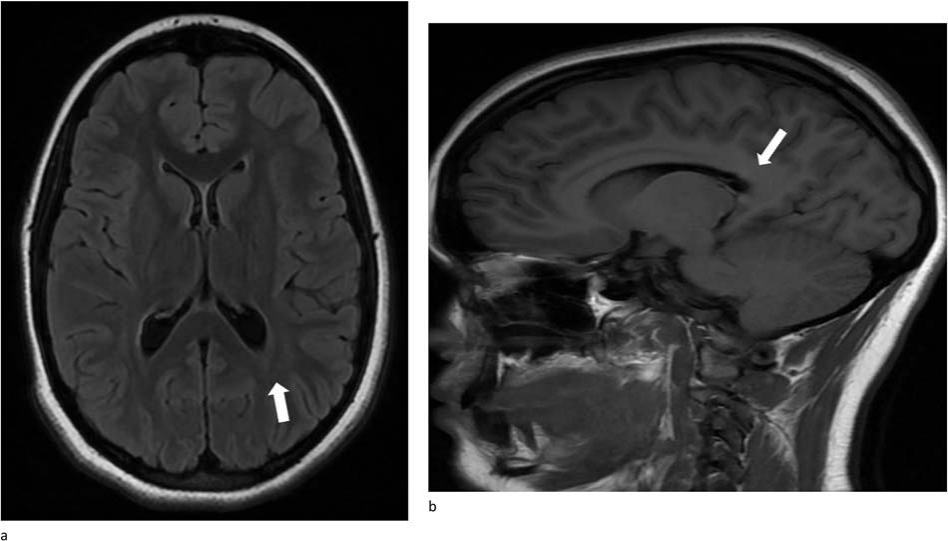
(a) and (b) Stable postsurgical findings compatible with gross total resection of left atrial and adjacent periatrial tumor. No MRI evidence of residual/recurrent mass, with normal ventricular size.
